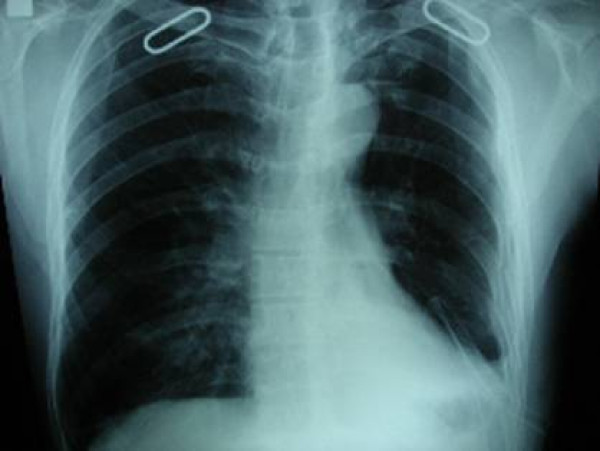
X-ray image of the other tube malposition patient. Despite the drain, retained haemothorax is observed.
